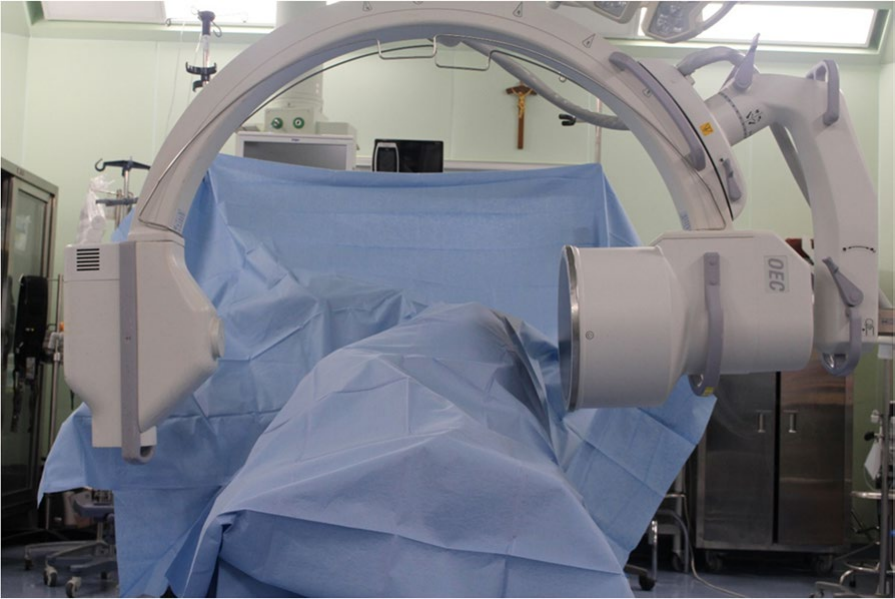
An actual example of an operating room for intraoperative radiography in this hospital. The patient is in a lateral decubitus position. A temporary sterile drape is over the patient. The X-ray by the C-arm intensifier is shot cross-table
